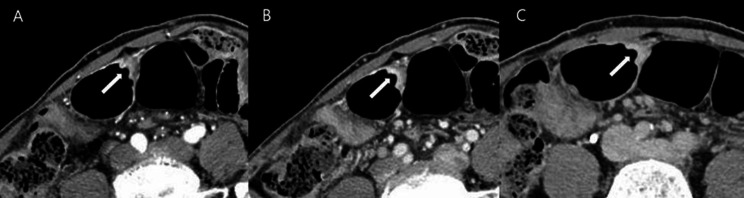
A patient with differentiated T2 stomach cancer. (A) In the arterial phase, the lesion (arrow) shows a double-layered wall with a non-enhancing outer layer, indicative of a negative dynamic CTTM sign. (B) In the portal phase, the lesion demonstrates a type II positive dynamic CTTM sign due to the thickened and higher enhancement of the outer low-density layer (arrow) compared to the normal gastric outer layer. (C) In the delayed phase, the lesion shows single-layer transmural enhancement (arrow), representing a type I positive CT transmural sign. The CTTM pattern is classified as 0-1-1
